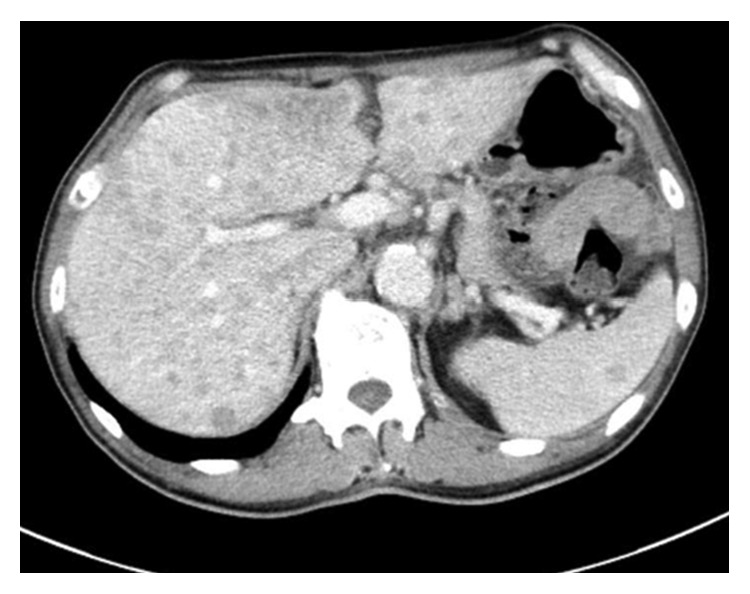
Abdominal contrast-enhanced CT shows multiple low-density areas, hepatomegaly, and metastasis to the spleen.
